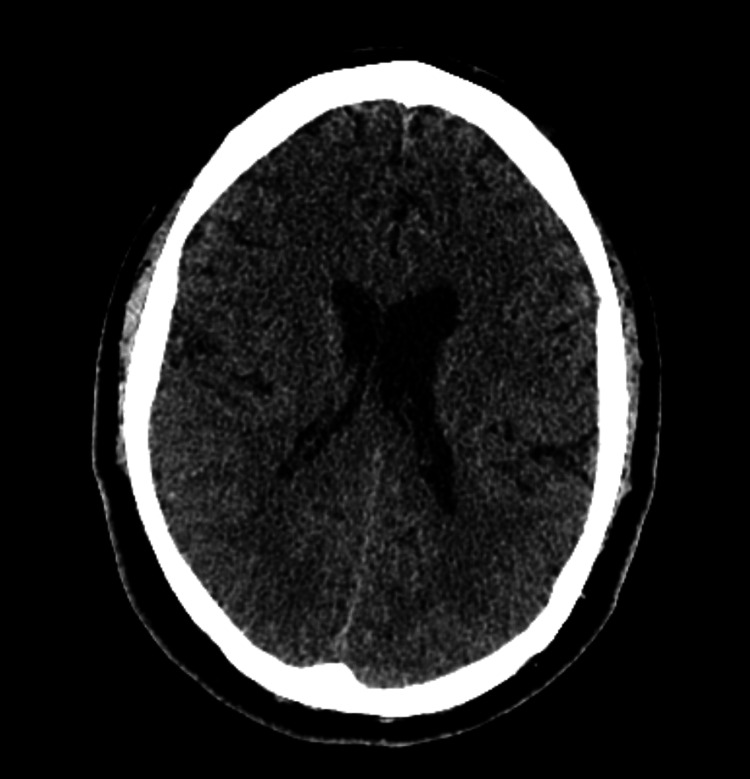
Non-contrast CT head demonstrating no acute intracranial process. Axial images show no evidence of territorial infarct, intracranial hemorrhage, mass effect, midline shift, or hydrocephalus. Ventricular size is within normal limits, and basal cisterns are patent. Paranasal sinuses and mastoid air cells appear clear.
